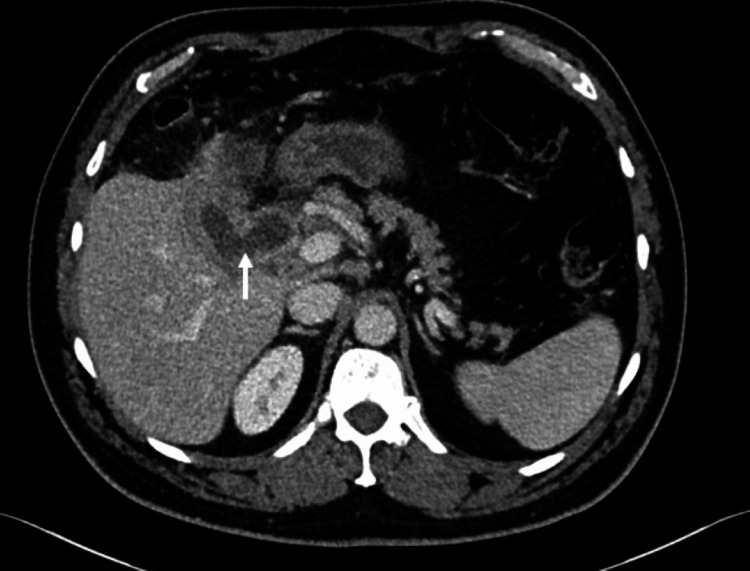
Axial portal venous CT demonstrates a fistula between gallbladder and duodenum (white arrow).
